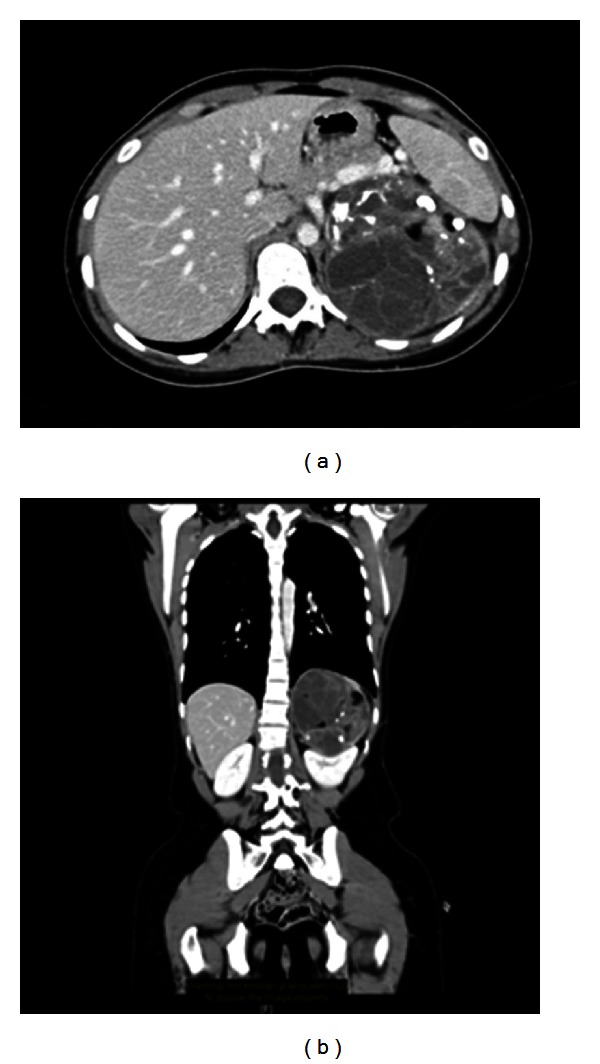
Abdominal contrast-enhanced computed tomography (CT) scan. (a) Cross-sectional (transverse) view: showing a large lesion in the region of left adrenal gland measuring 9.0 × 9.2 × 10.8 cm and demonstrating multiple cystic spaces with thin septations as well as multiple areas of fatty collections and coarse calcifications. (b) Coronal (frontal) view: showing displacement of left kidney inferiorly.
